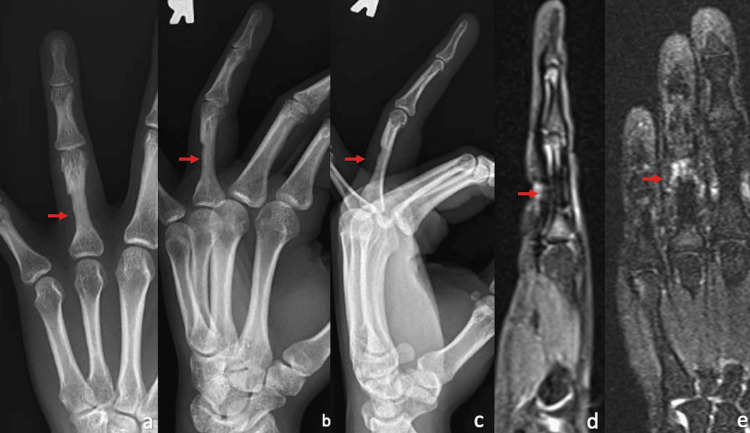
Radiographs (a-c) and MRI (d, e) demonstrating proximal phalanx bone resorption and osteitis (indicated by the red arrows) 16-months status post index pulley reconstruction with intact palmaris longus autograft.
